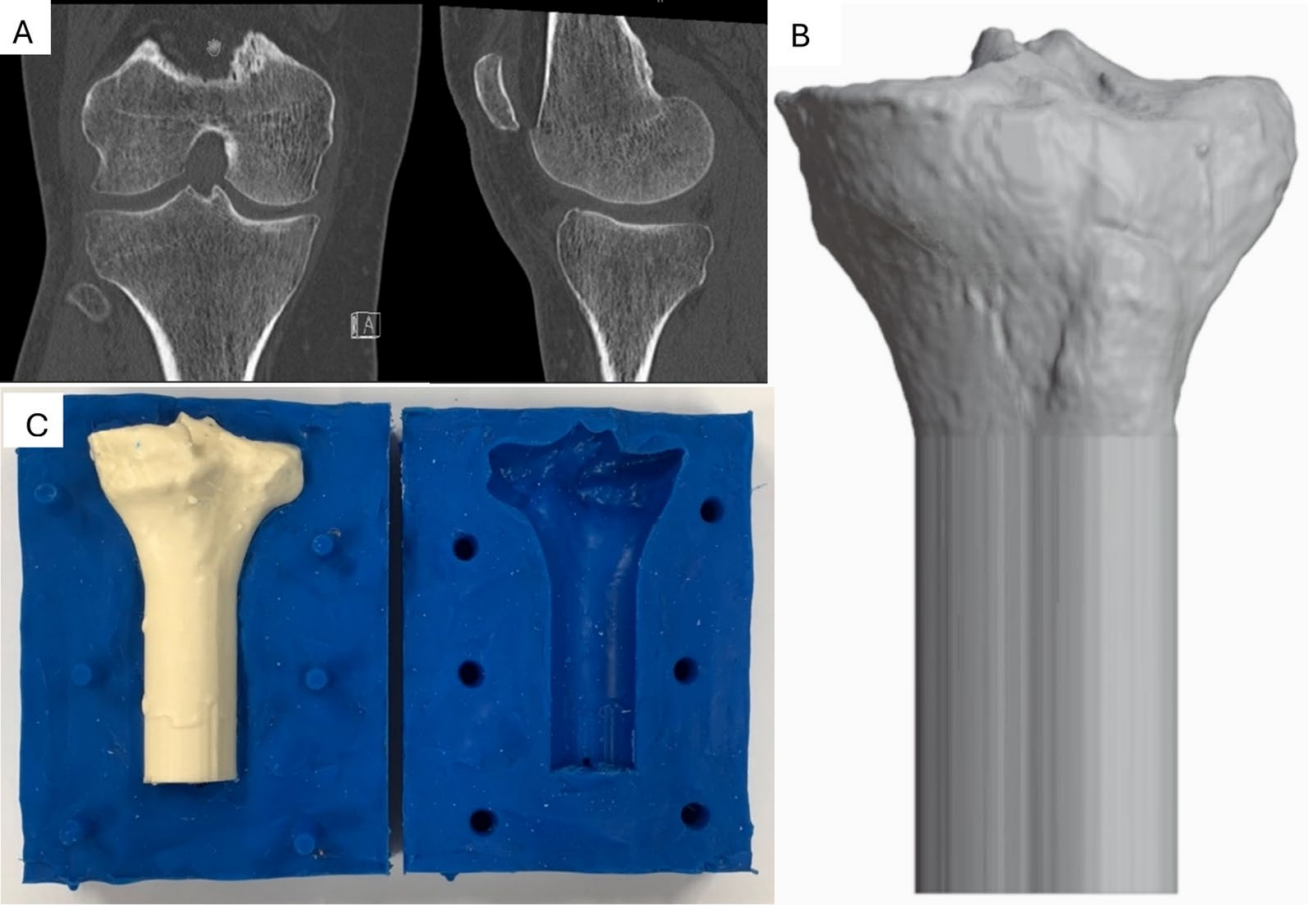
Initial CT scan of the proximal tibia in the coronal and sagittal plane (A). Modified 3D model (B). The tibial shaft was lengthened extra-anatomically for stable fixation during biomechanical testing. The 3D-printed tibial model was used to create silicon molds for the cancellous and the cortical model, respectively (C)
